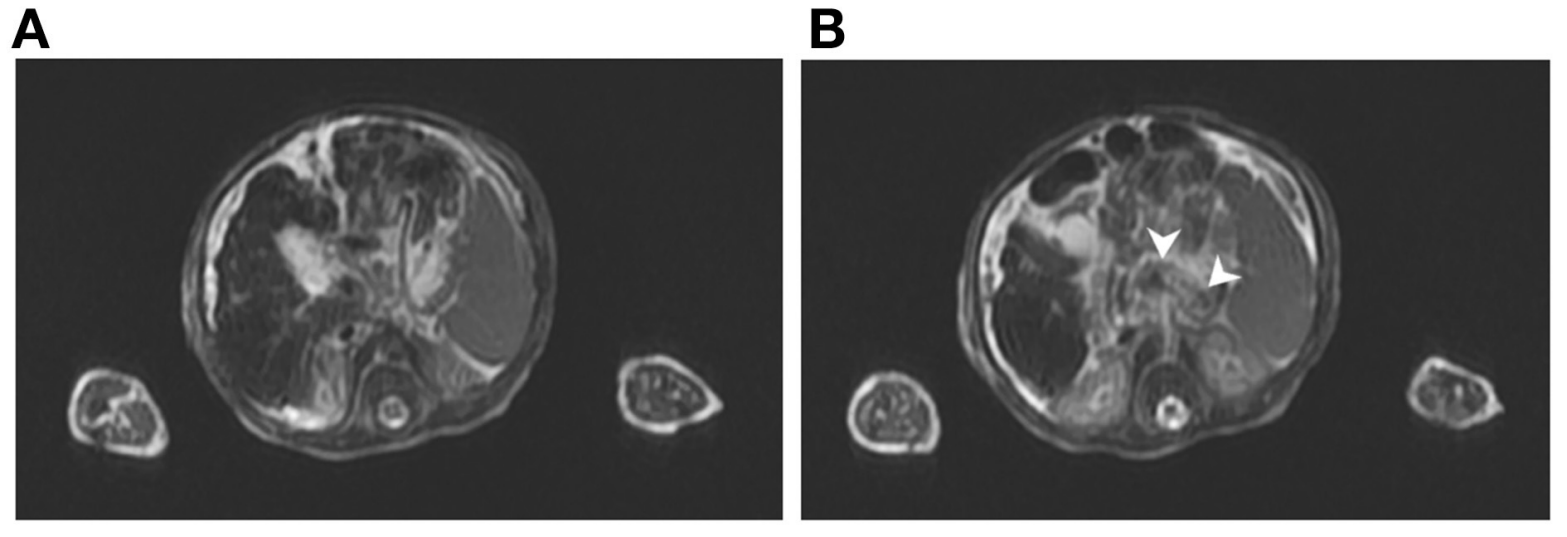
T2-weighted magnetic resonance imaging of case: (A) Siderosis and cirrhosis of liver and (B) metal-induced susceptibility artifact, indicating siderosis of pancreatic body (arrowhead).
